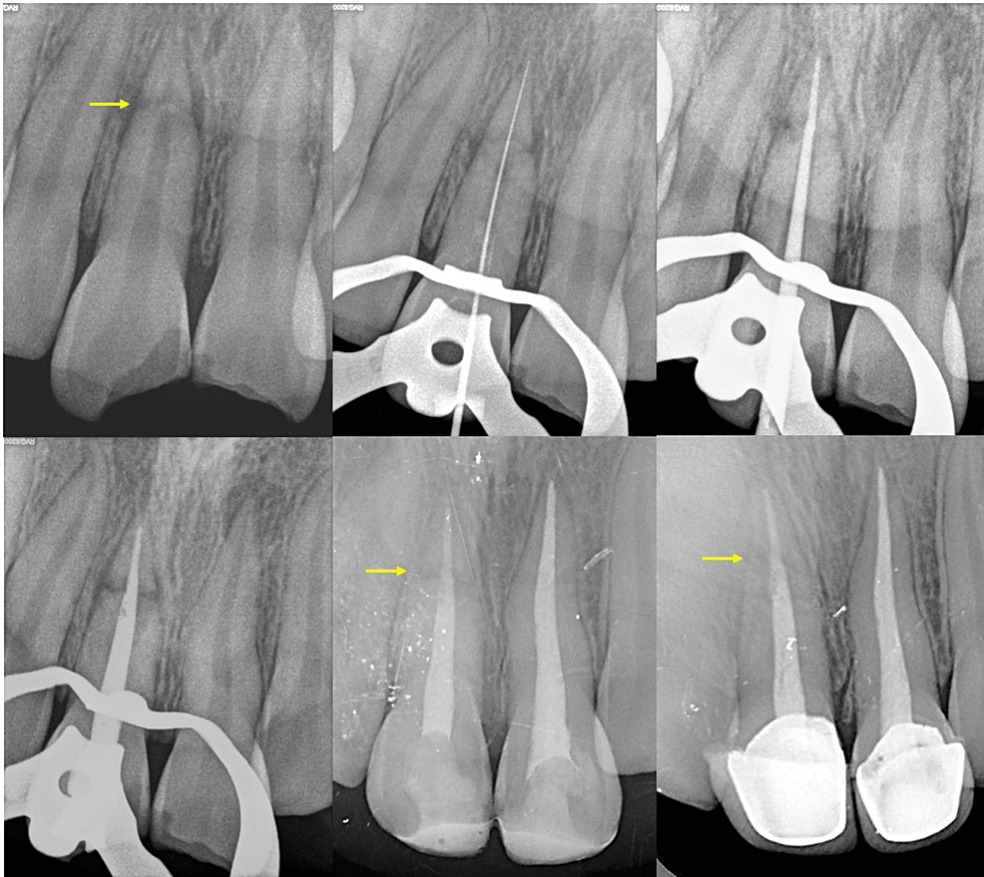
(A) Pre-operative radiograph showing HRF in 11 as represented by the yellow arrow; (B) working length determination; (C) master cone selection; (D) obturation of both fragments till the apex; (E) post-obturation restoration and 12 months follow-up. Yellow arrow shows healing of the inter-fragmentary space. (F) Placement of prosthesis and 24 month follow-up. Yellow arrow shows complete healing and hard tissue repair.
